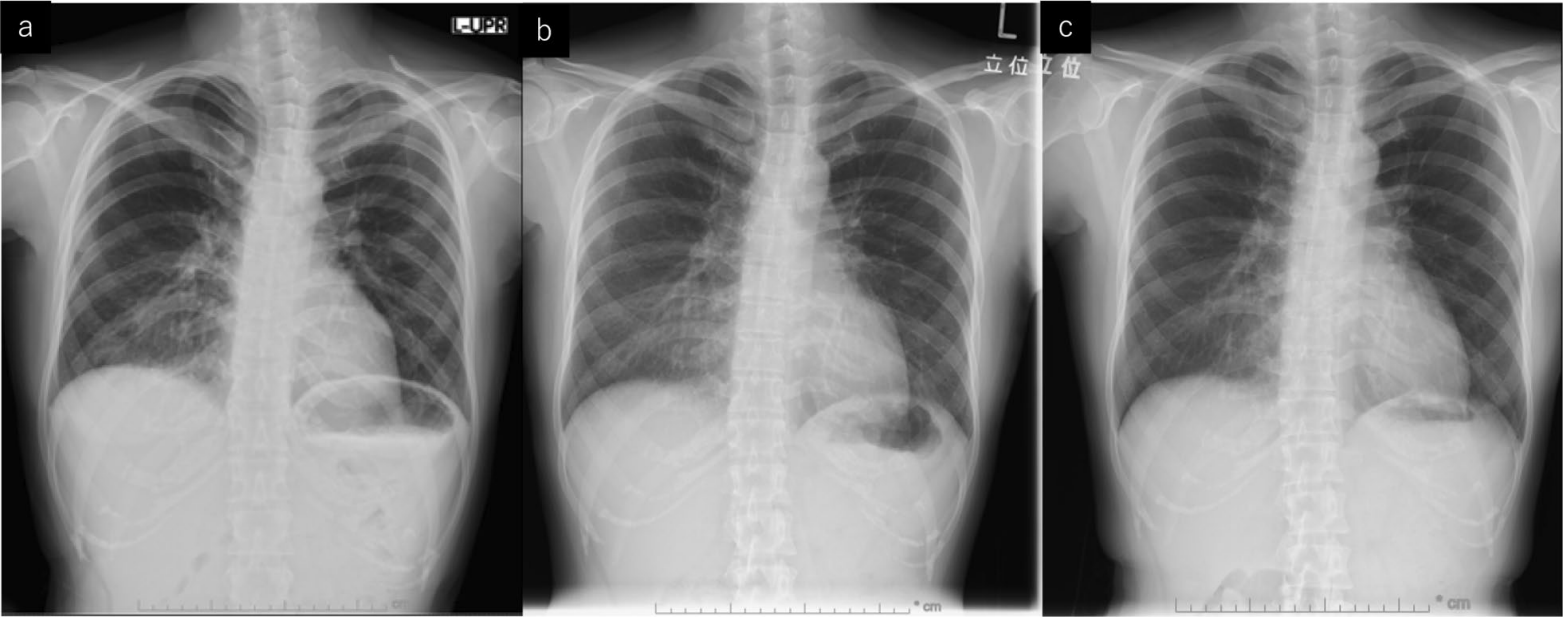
Chest X-ray findings. a X-ray on day of COVID-19 diagnosis indicating infiltrative shadows in the peripheral lesion of both lungs. b X-ray after 2 weeks from discharge showing benign organized pneumonia in both lungs. c X-ray 3 months after discharge showing the dissipation of organized pneumonia
